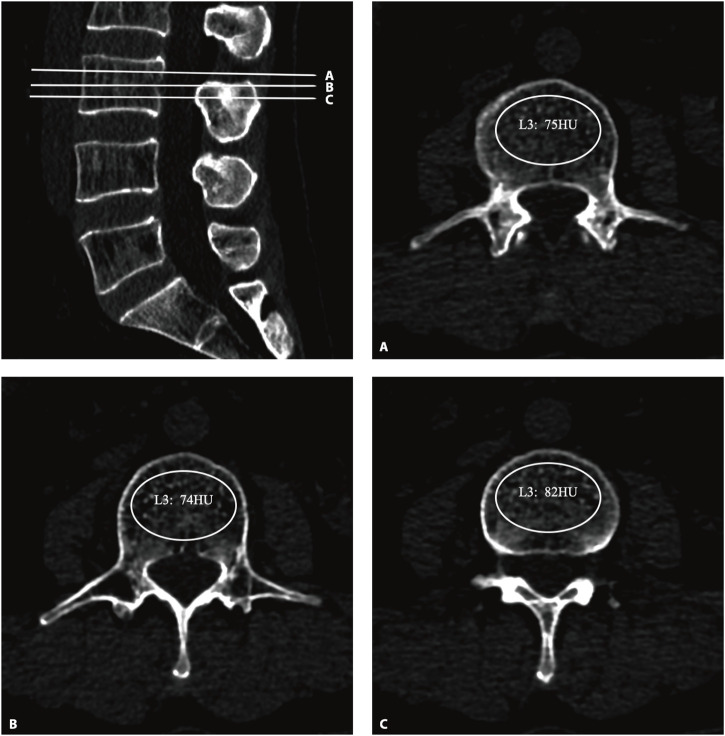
The HU values were derived from CT. An oval region of interest is positioned on the axial plane of the vertebrae (A–C), with the HU value being automatically calculated by the image archiving and communication system. HU, Hounsfield unit.
